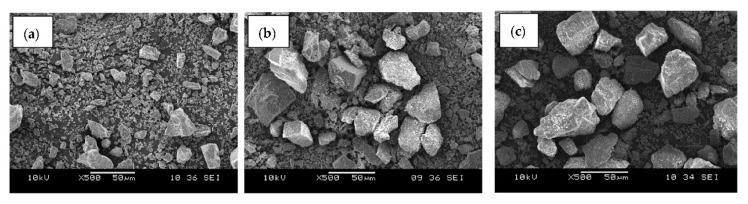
SEM images of dolomite that has been tip-ultrasonicated for (a) 2 h, (b) 3 h, and (c) 5 h at ×500 magnification.
